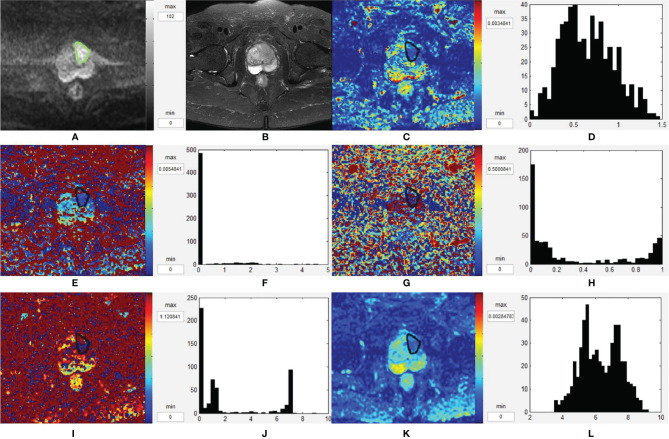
Representative images from a 76-year-old PCa patient with Gleason score of 3 + 4, as outlined on the images. (A–L) Axial DWI (*b* = 1,500 s/mm^2^), axial T2WI, *D* map, histogram of *D*, *D map, histogram of *D, *f* map, histogram of *f*, *K* map, histogram of *K*, ADC map, and histogram of ADC, respectively. PCa, prostate cancer; T2WI, T2-weighted images; DWI, diffusion-weighted images; ADC, apparent diffusion coefficient.
